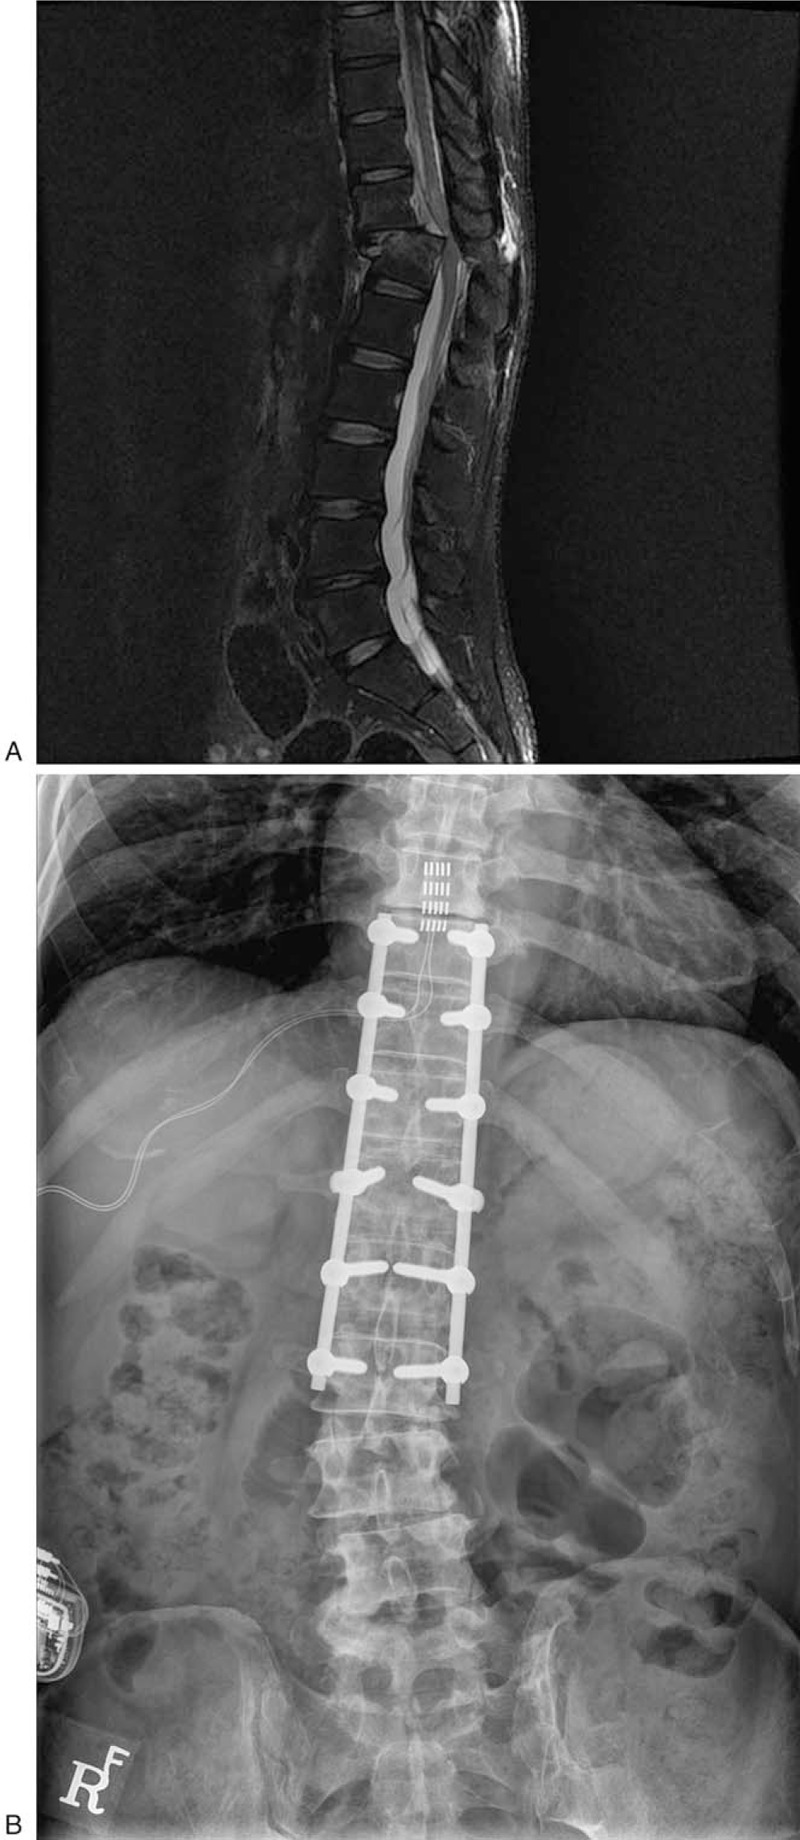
MR and pain film images obtained in Case 1. (A) Preoperative MRI showing a T12 bursting fracture combined with dislocation; (B) Plain x-ray showing an SCS electrode placed at the T9 level and a permanent rechargeable neurostimulation system.
